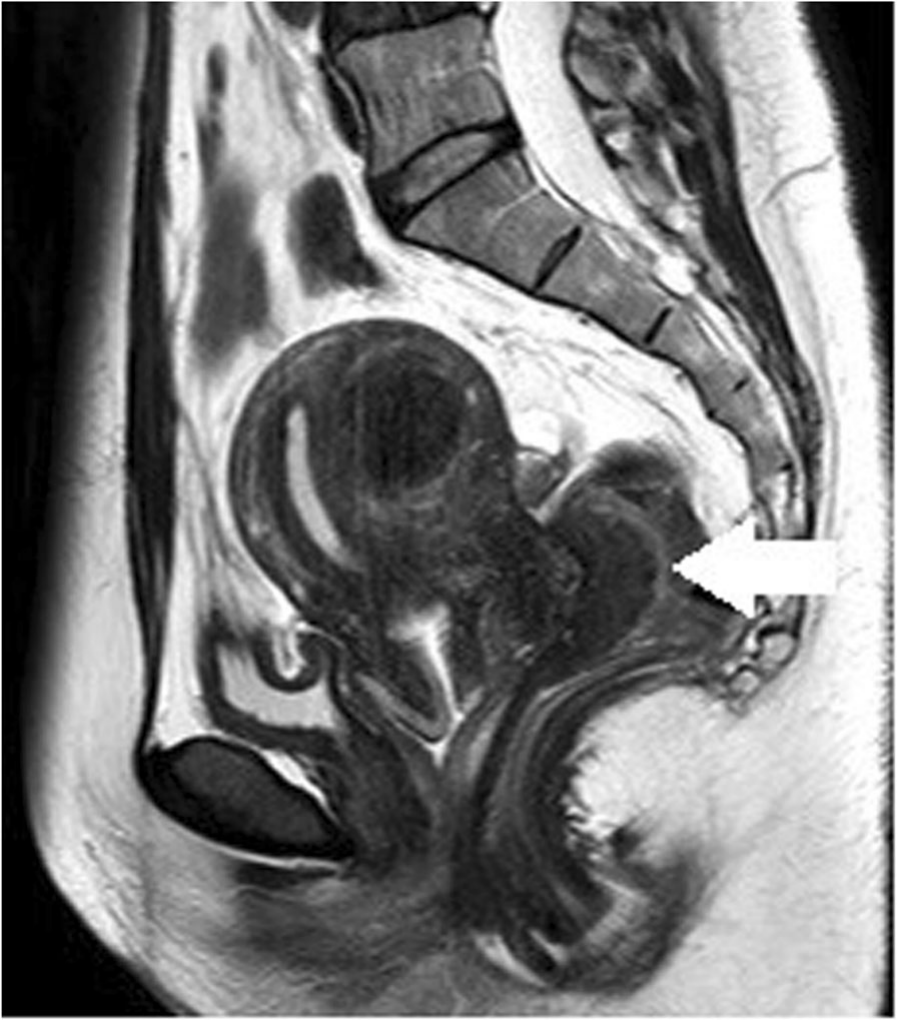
Magnetic resonance imaging (MRI) shows a 40-mm mass on the anterior wall of the rectum
